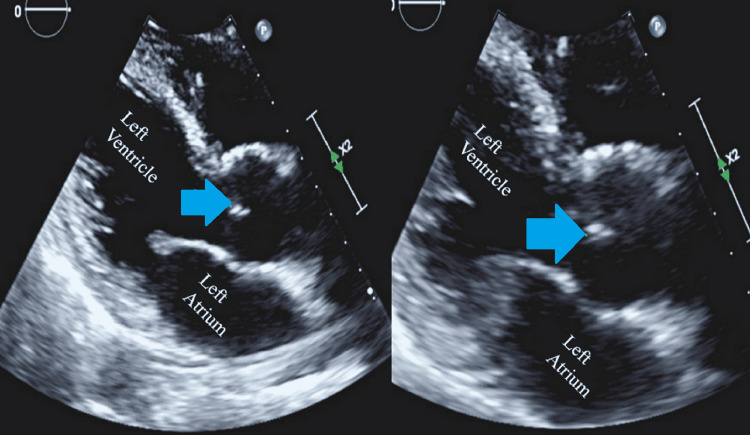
Transthoracic echocardiogram in parasternal long axis view showing vegetation on the right coronary cusp of the aortic valve (blue arrows)
